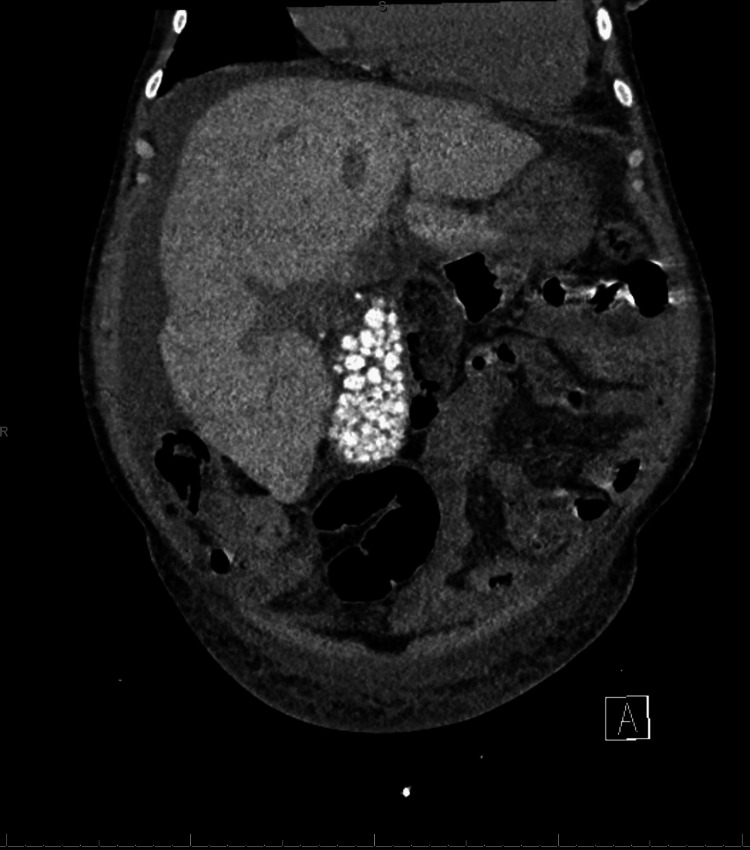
Abdominal CT, coronal view, demonstrating hepatomegaly and cholelithiasis
